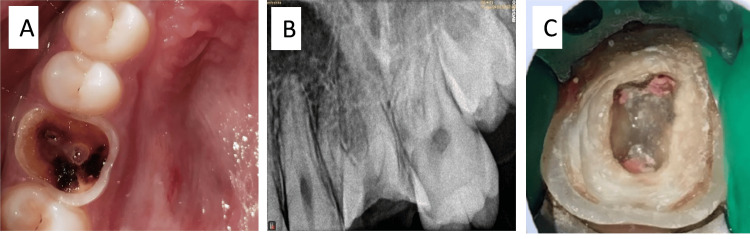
(A) Preoperative clinical image. (B) Preoperative radiograph. (C) After obturation.
